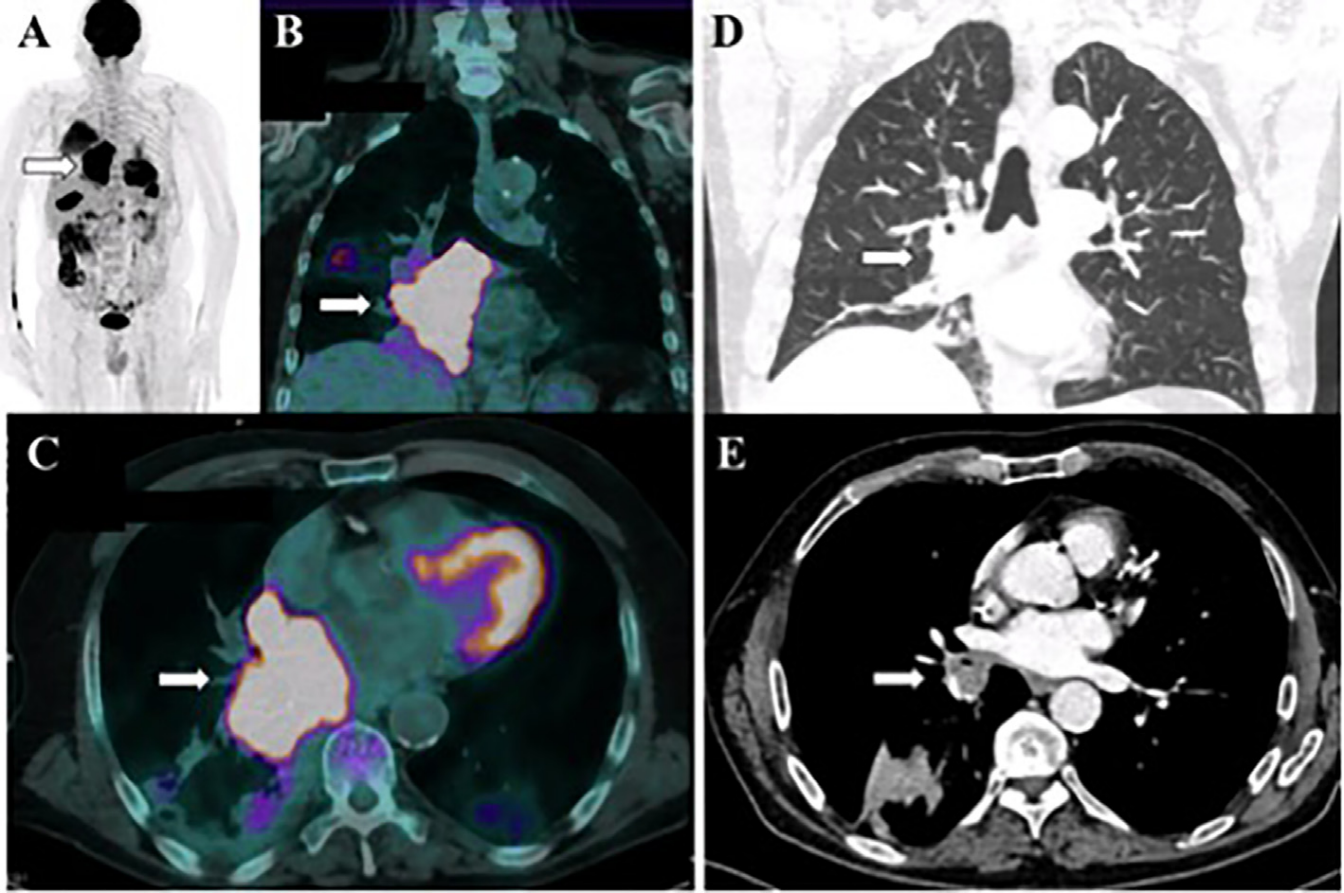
Case 2 (A-C) PET CT at diagnosis shows an intensely hypermetabolic mass at right lower lobe adjacent to mediastinum (white arrows). (D, E) CT image of the chest performed 16 months after diagnosis shows partial response of the primary lung tumor (white arrows).
